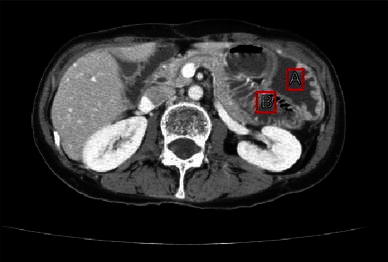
Abdominal computed tomography. Caliber changes are seen in the small bowel. *A* stomach; *B* ileum
